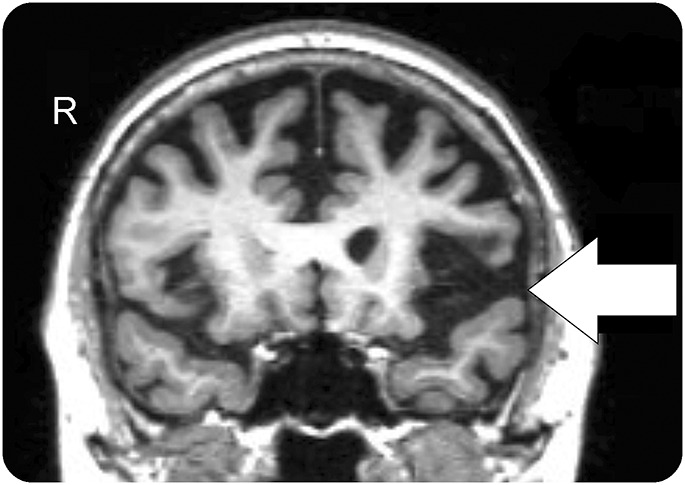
Magnetic resonance image Coronal volumetric T1-weighted MRI showing asymmetric atrophy of left insula and opercular inferior frontal gyrus (arrow), a pattern typical of nonfluent variant primary progressive aphasia.
